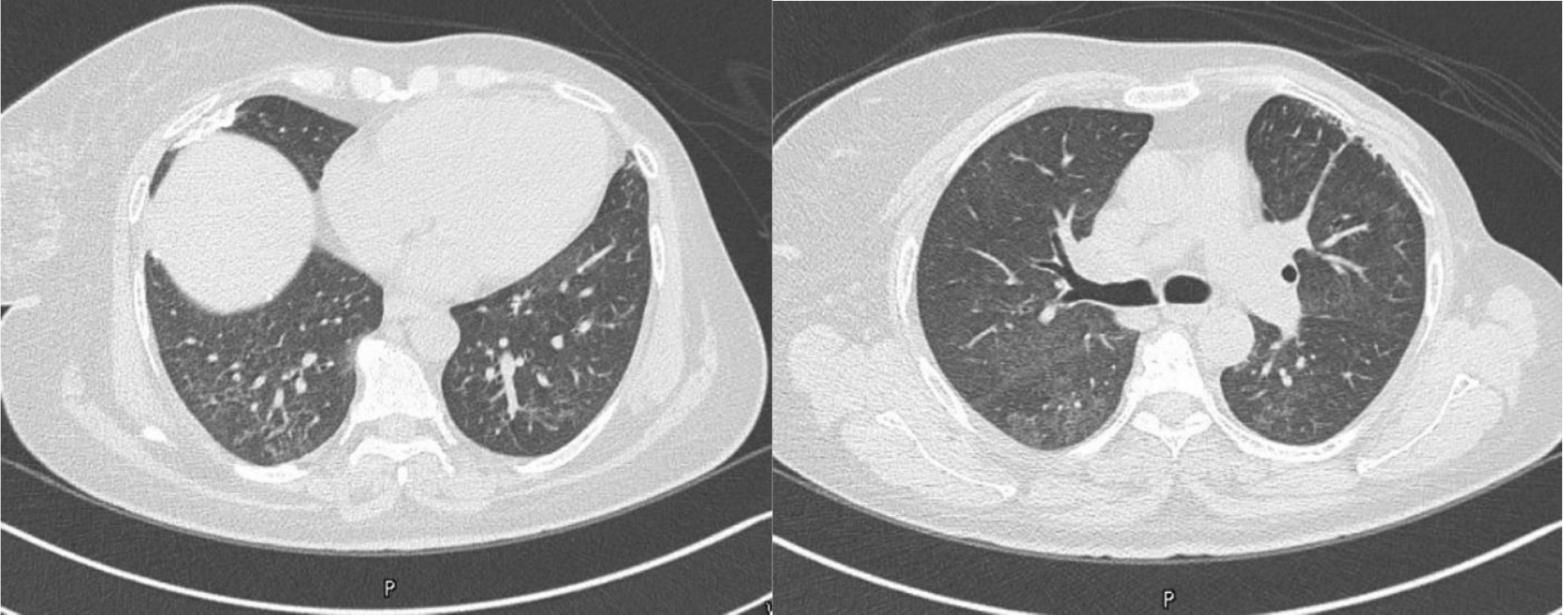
Chest CT scan showing appreciable regression of the previously reported bilateral consolidation, ground-glassing, and reticular patterns.
